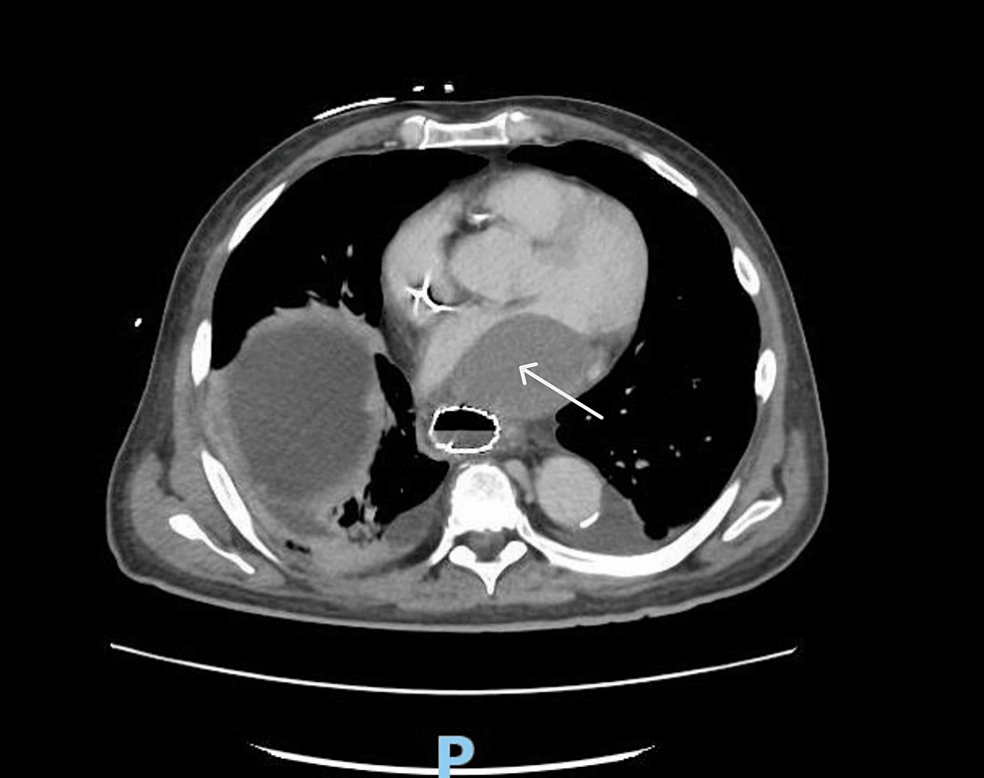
CT abdomen/pelvis with IV contrast with an ovoid thrombus versus mass/metastasis visualized in the left atrium
